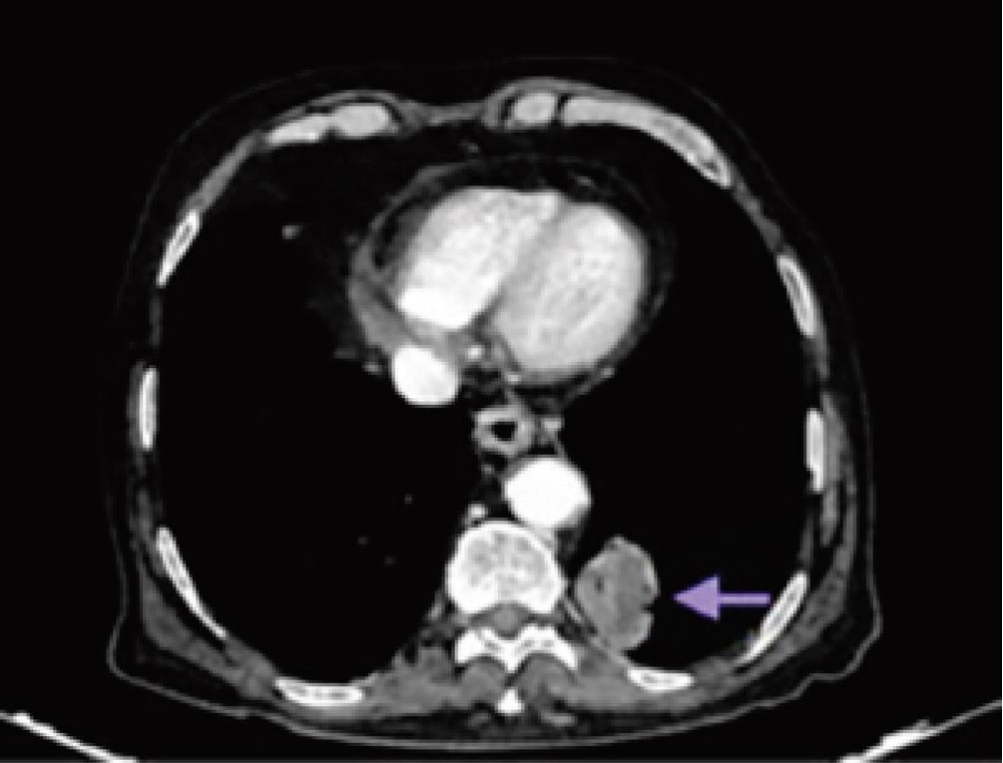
Contrast‐enhanced computed tomography of the chest showing an enhancing lesion with soft tissue attenuation (arrow) in the left lower lobe suggestive of lung cancer.
